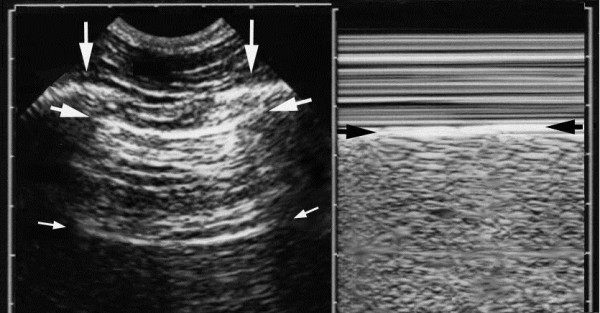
Normal lung surface. Left: Scan of the intercostal space. The ribs (vertical arrows). Rib shadows are displayed below. The pleural line (upper, horizontal arrows), a horizontal hyperechoic line, half a centimeter below the rib line in adults. The proportions are the same in neonates. The association of ribs and pleural line make a solid landmark called the bat sign. The pleural line indicates the parietal pleura in all cases. Below the pleural line, this horizontal repetition artifact of the pleural line has been called the A-line (lower, small horizontal arrows). The A-line indicates that air (gas more precisely) is the component visible below the pleural line. Right: M-mode reveals the seashore sign, which indicates that the lung moves at the chest wall. The seashore sign therefore indicates that the pleural line also is the visceral pleura. Above the pleural line, the motionless chest wall displays a stratified pattern. Below the pleural line, the dynamics of lung sliding show this sandy pattern. Note that both images are strictly aligned, of importance in critical settings. Both images, i.e., lung sliding plus A-lines make the A-profile (when found at the anterior chest wall). They give basic information on the level of capillary pressure. Extract from “Whole body ultrasonography in the critically ill” (2010 Ed, Chapter 14), with kind permission of Springer Science.
